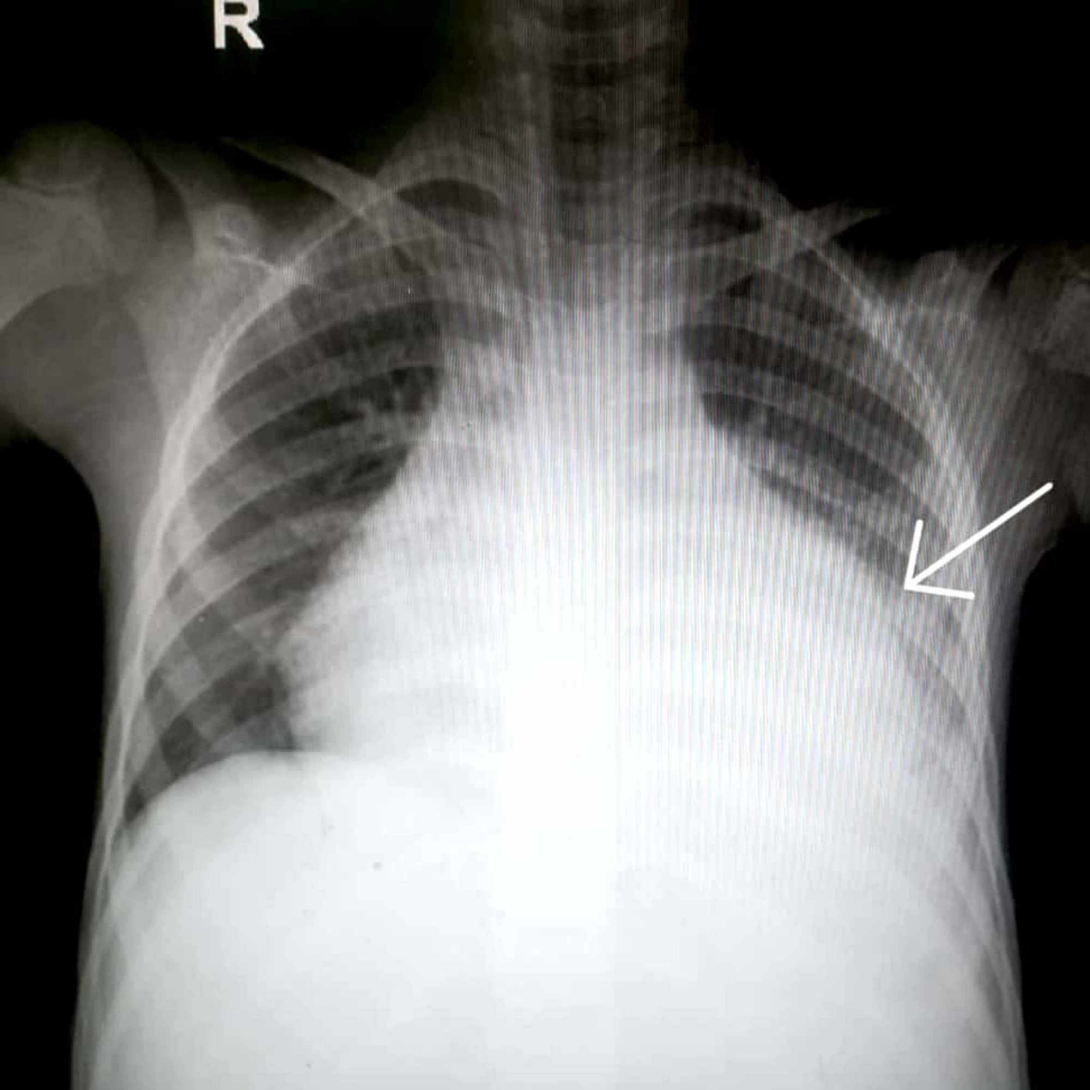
Chest X-ray showing enlargement of the cardiac silhouette with clear lung fields
